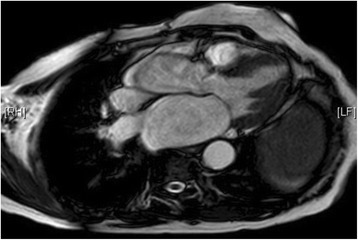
Cardiovascular magnetic resonance imaging showing apical left ventricular hypertrophy
